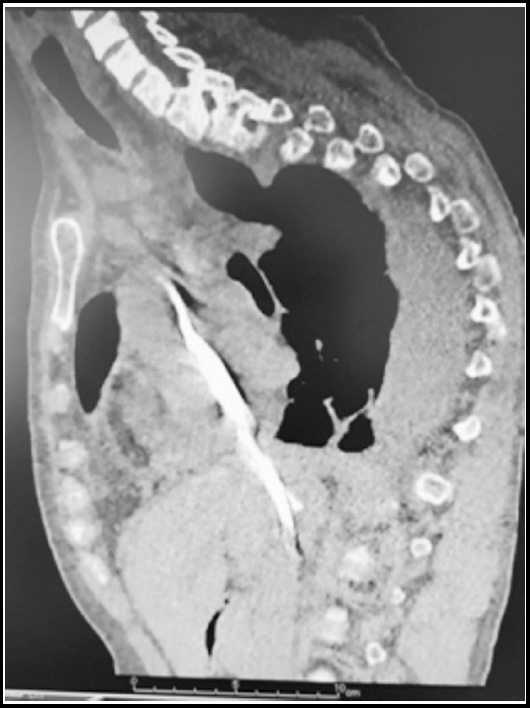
Sagittal oblique reformatted images of the non Contrasted computed tomography scan. The catheter in the jugular vein extends towards the inferior vena cava and into the hepatic vein.
